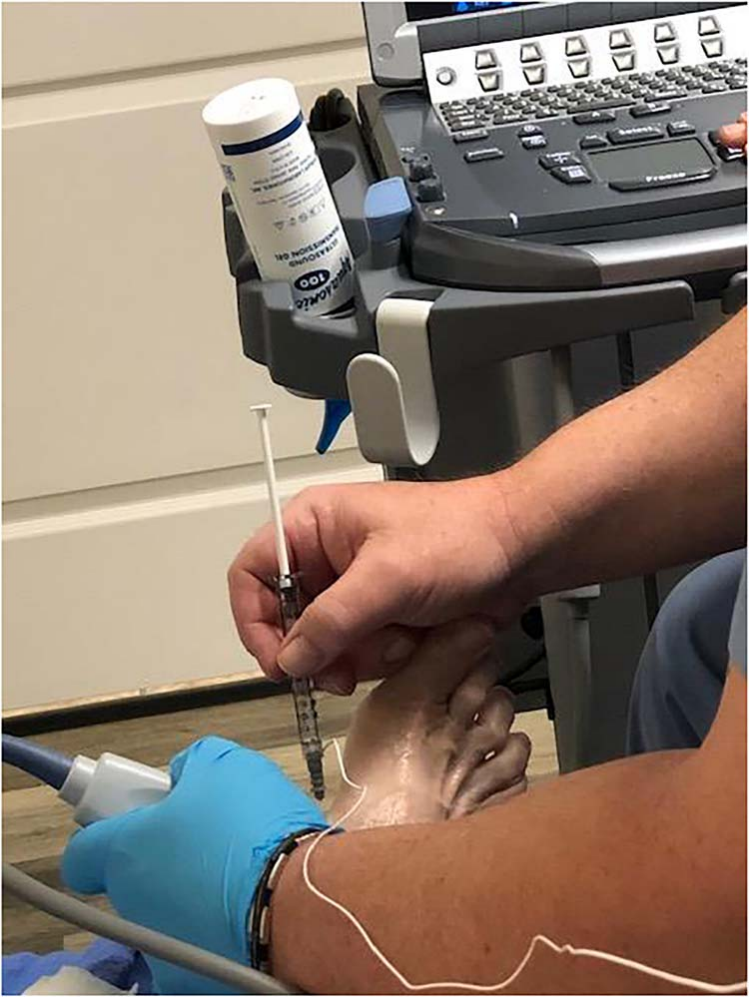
Ultrasound guided botulinum toxin injection. 30-gauge needle was used to inject the Botulinum toxin into the abductor hallucis brevis muscle belly.
